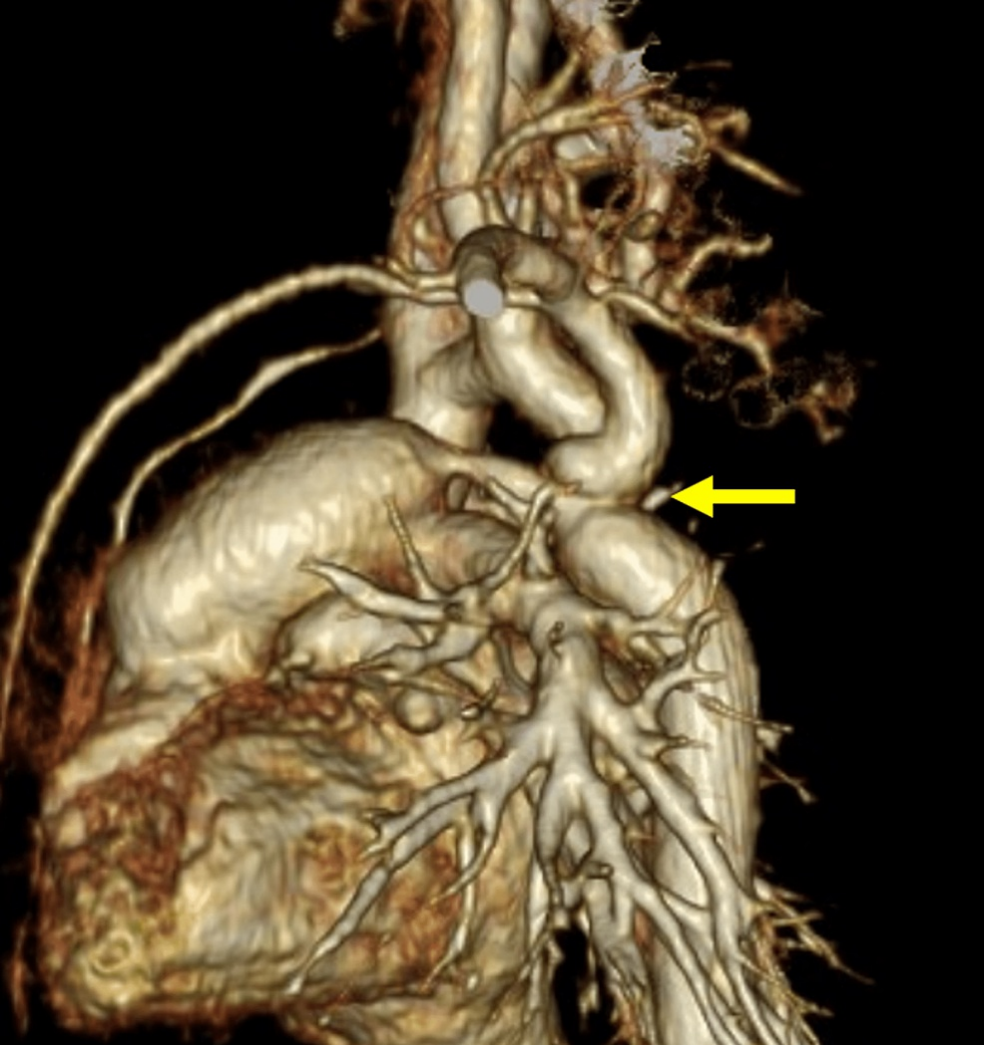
Enhanced computed tomography (arrow) Enhanced computed tomography on postnatal day 4 showed a narrowing isthmus.
